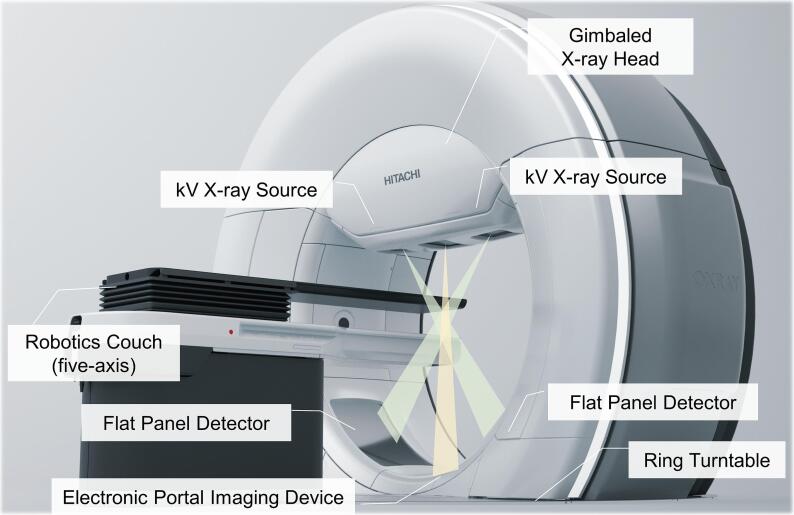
Appearance of the OXRAY system. The OXRAY system includes a gimbaled X-ray head, a dual kV X-ray imaging subsystem, an electronic portal imaging device, and a robotic treatment couch with five degrees of freedom (i.e., three translational axes and two rotational axes, pitch, and roll) for patient set-up correction.
